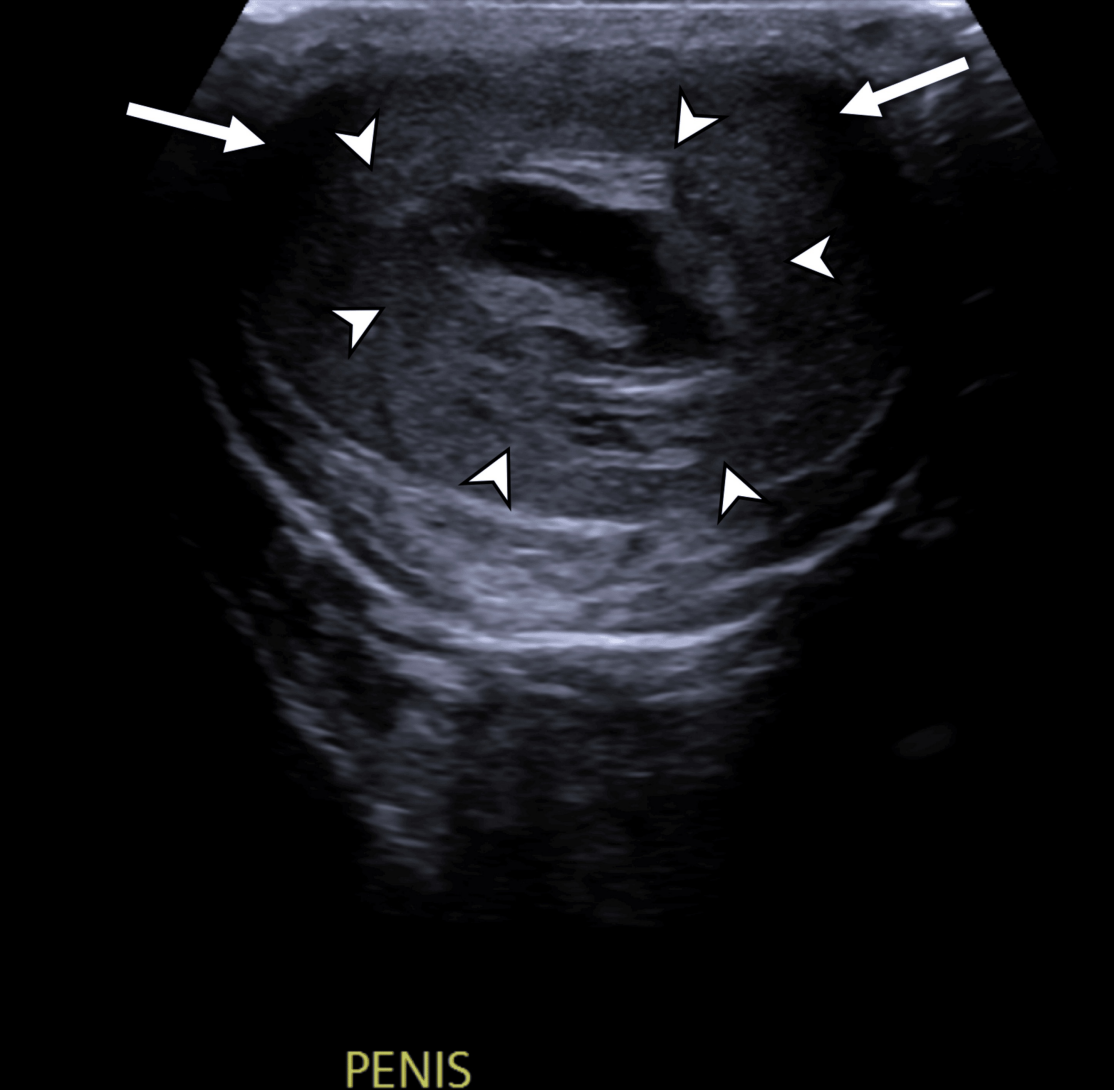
Greyscale ultrasound of the penis in transverse section view Note the central haematoma (white arrowheads) surrounded by penile corpora (white arrows).
